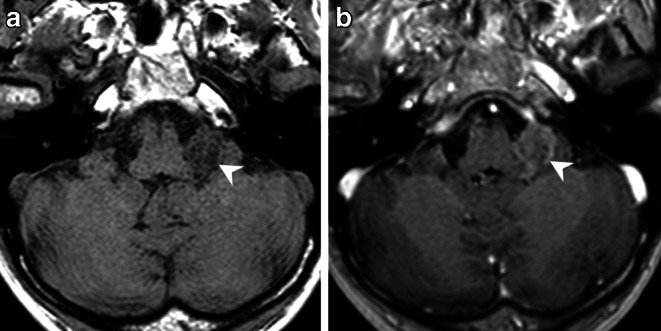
On an axial native T1-weighted image (a) the lesion (*arrowhead*) appears hypointense when compared to the cerebellar tissue. On an axial (b) T1-weighted images after administration of gadolinium the lesion shows mild partial capsular enhancement of contrast (b, *arrowhead*)
